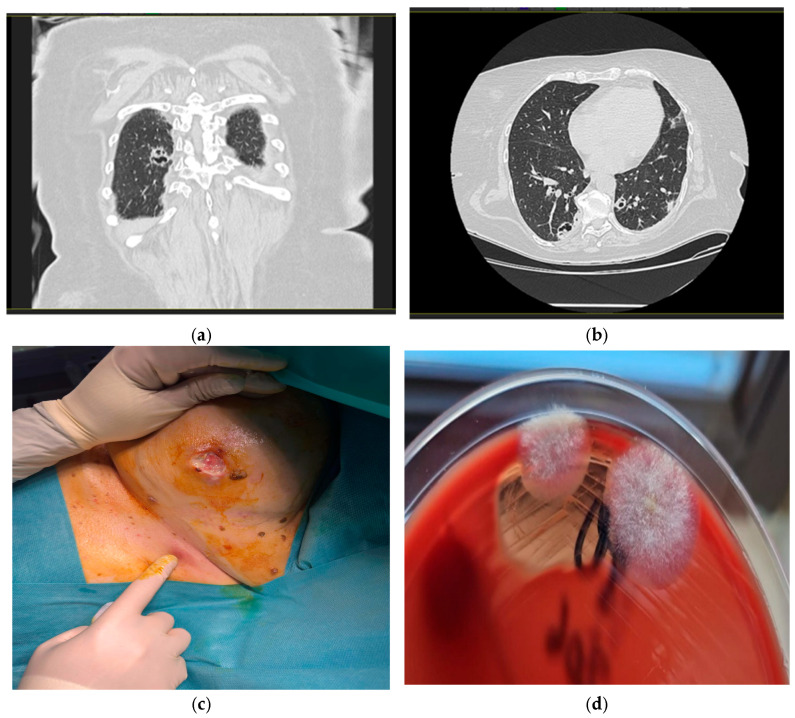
Radiologic and microbiologic features of cavitary lesions. (a) Coronal CT image showing a cavitary lesion in the right upper lobe of the lung; (b) axial CT image of a cavitary lesion in right lower pulmonary lobe; (c) fistulized nodular lesion in the right submammary region; (d) sample culturing on Columbia agar with ram blood showed the white and cottony colonies.
